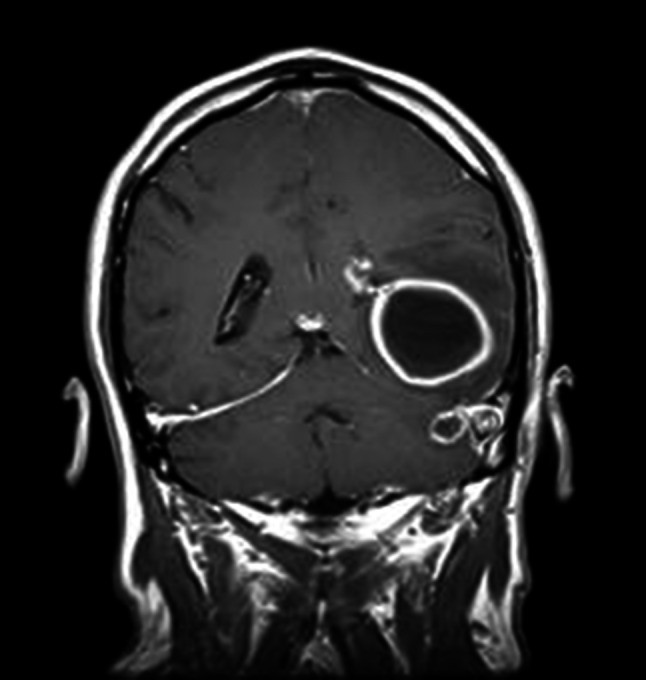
MRI image of the head in coronal plane shows the circular pathological mass in the left temporal lobe-abscess (the 52-year-old patient)
